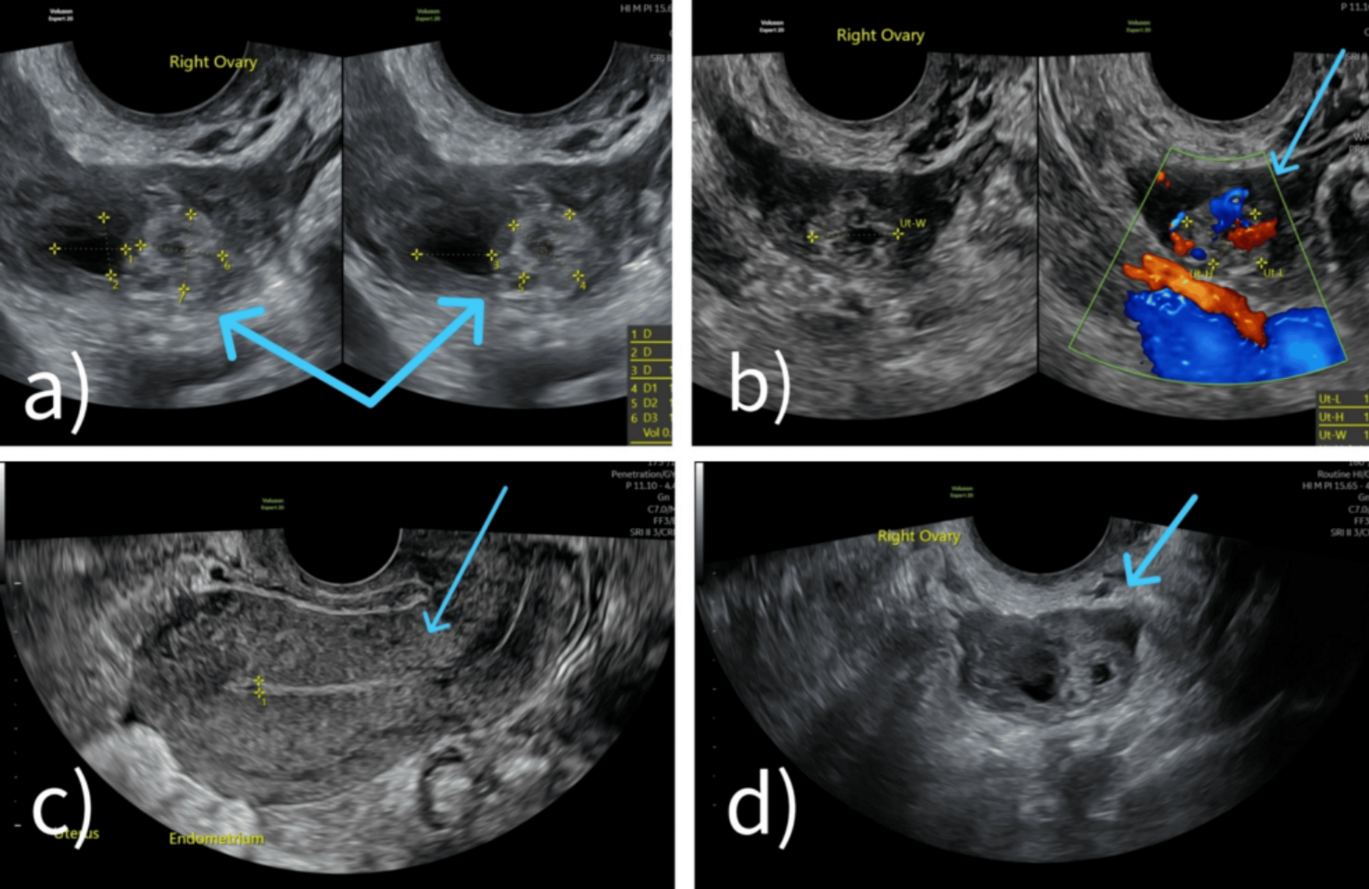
Transvaginal ultrasound images of Case 1 (a) Right ovary, where the double arrow indicates the cystic area suspicious for ectopic implantation vs. corpus luteum. (b) Right ovary with color Doppler showing vascular flow around the lesion, also referred to as the ring of fire sign. (c) Longitudinal view of the uterus with thin endometrium and no intrauterine gestational sac. (d) Right ovary, where the arrow points to a complex cystic mass with mixed echogenicity, consistent with either a hemorrhagic corpus luteum and/or ovarian ectopic tissue
